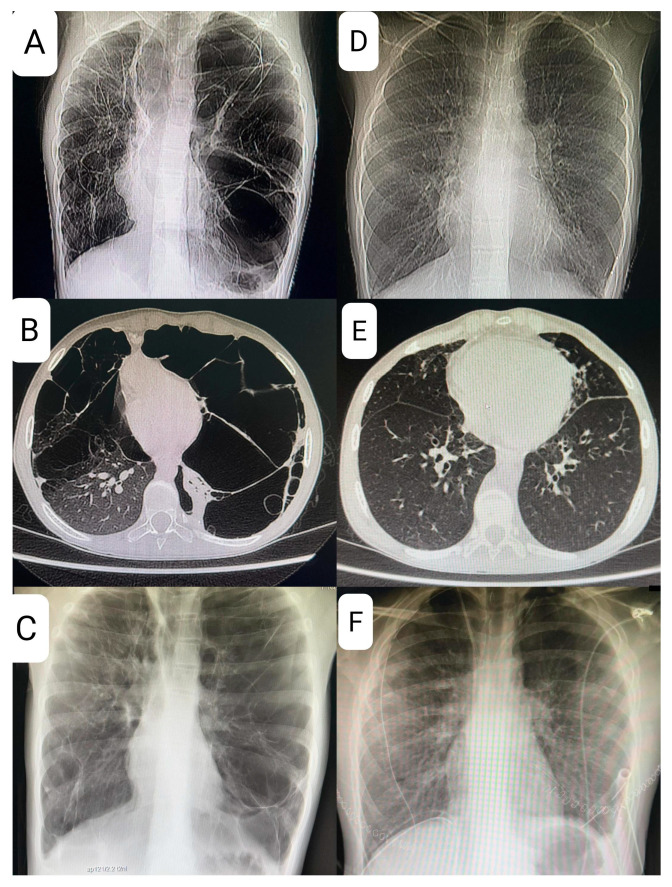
(A–C)—preoperative images—large cysts and shift of the mediastinum to the right; (A): CT topogram, (B): CT transverse section, (C): X-ray p–a; (D–F)—postoperative images—normal lung tissue; (D): CT topogram, (E): CT transverse section, (F): X-ray.
